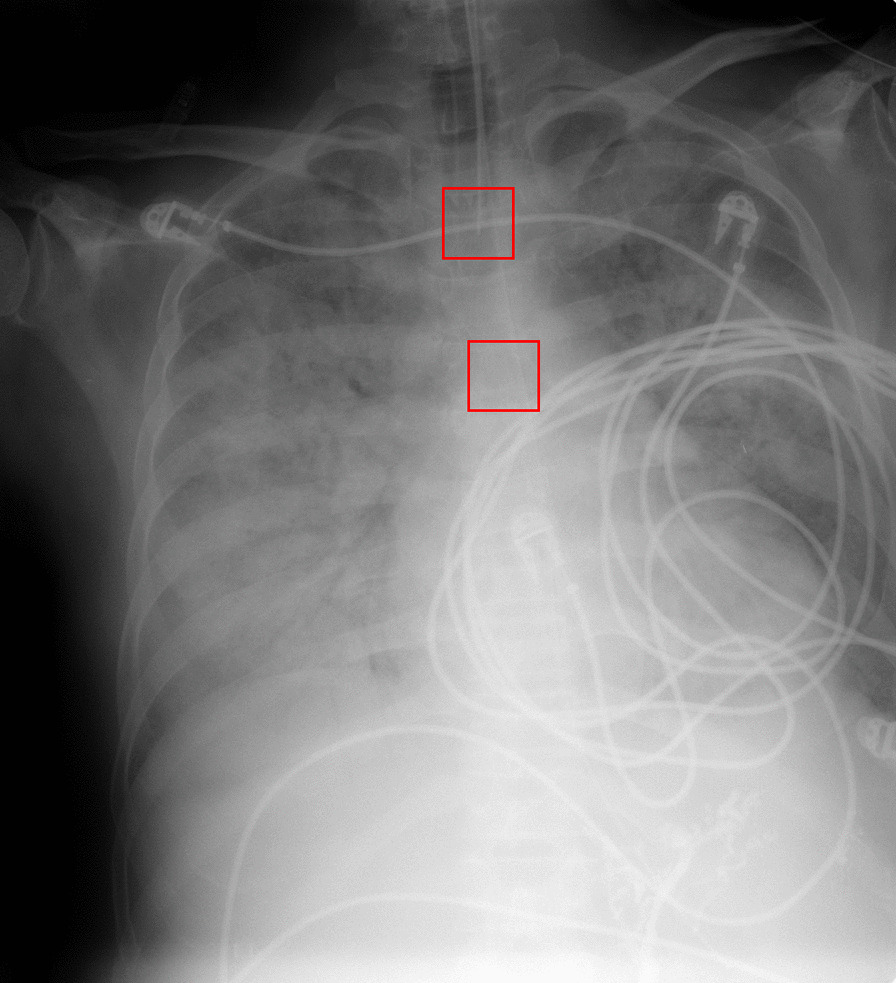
Bounding boxes around the carina and the endotracheal tube tip on a chest radiograph
